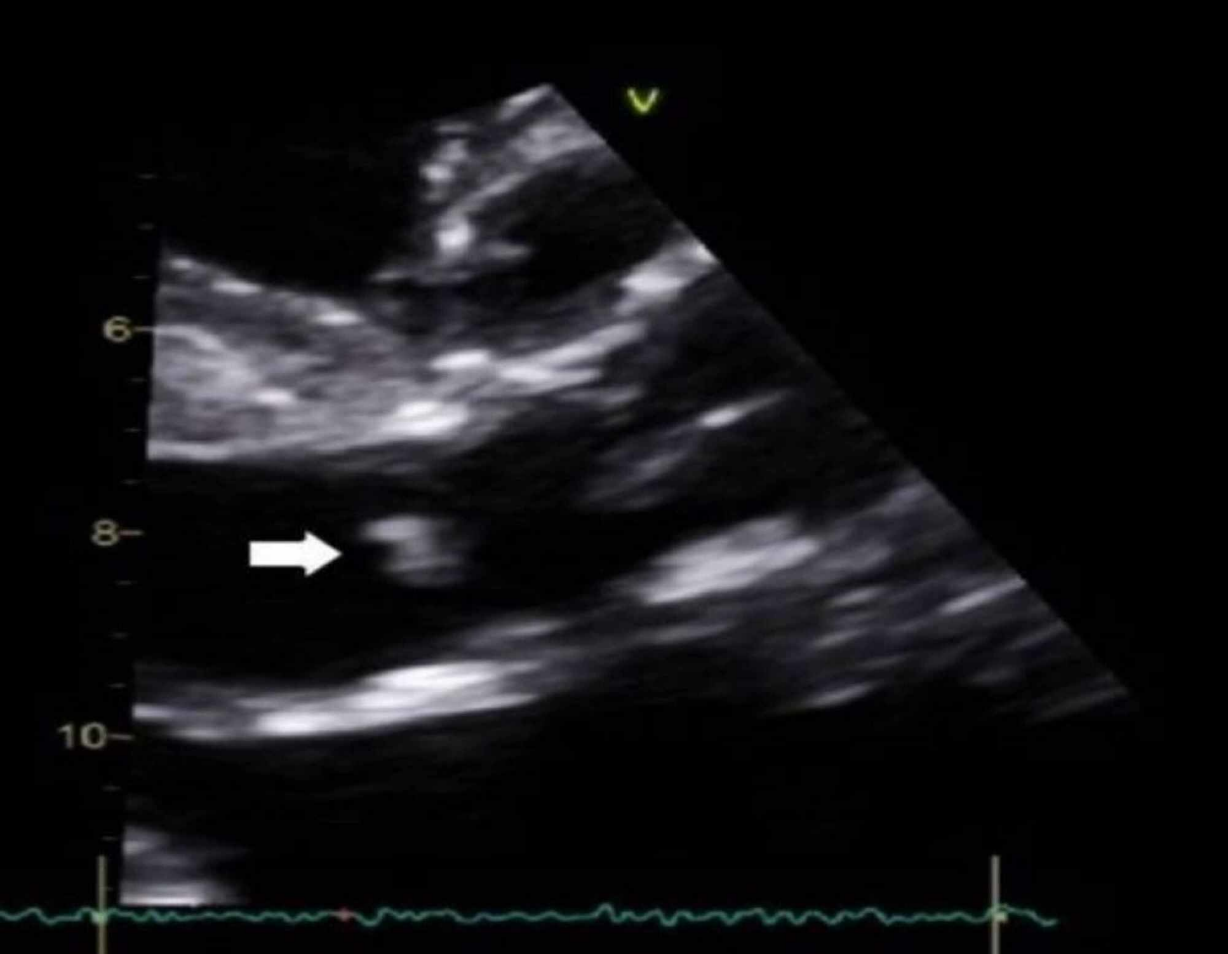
Aortic valve endocarditis as seen on the transthoracic echocardiogram
